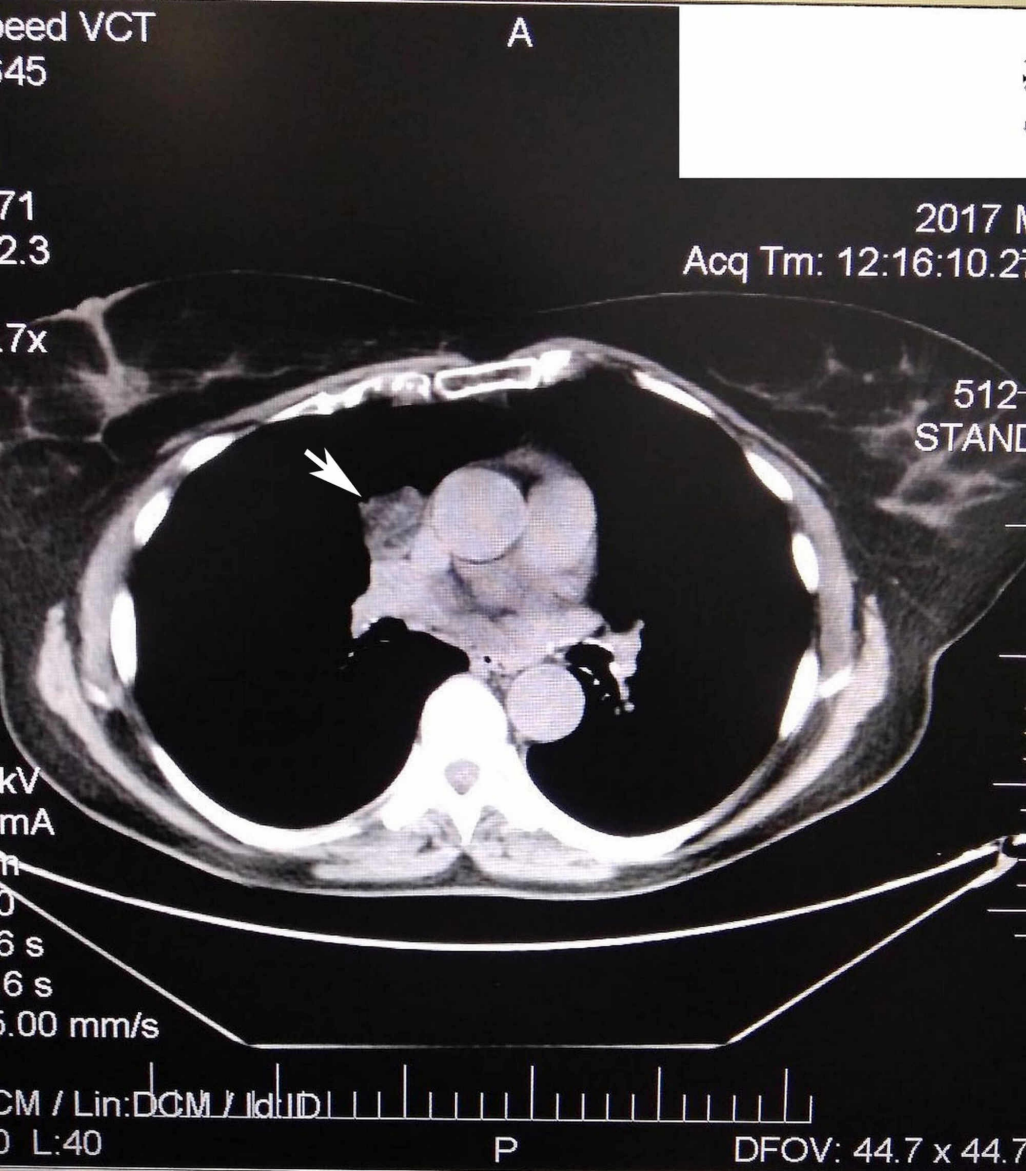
CT-scan showing the oval mass in the right side medial mediastinum
